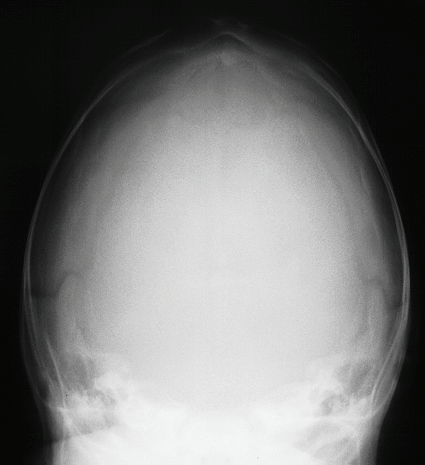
Skull X-ray in scaphocephaly AP imaging
